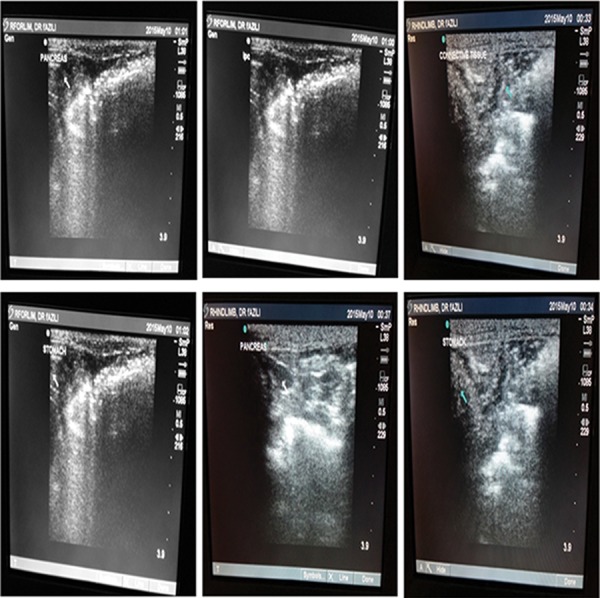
Ultrasound images for assessment of scaffold location after implantation in caudal of stomach. White arrow shows site of implantation near to the pancreas as a heteroechogenic mass.
